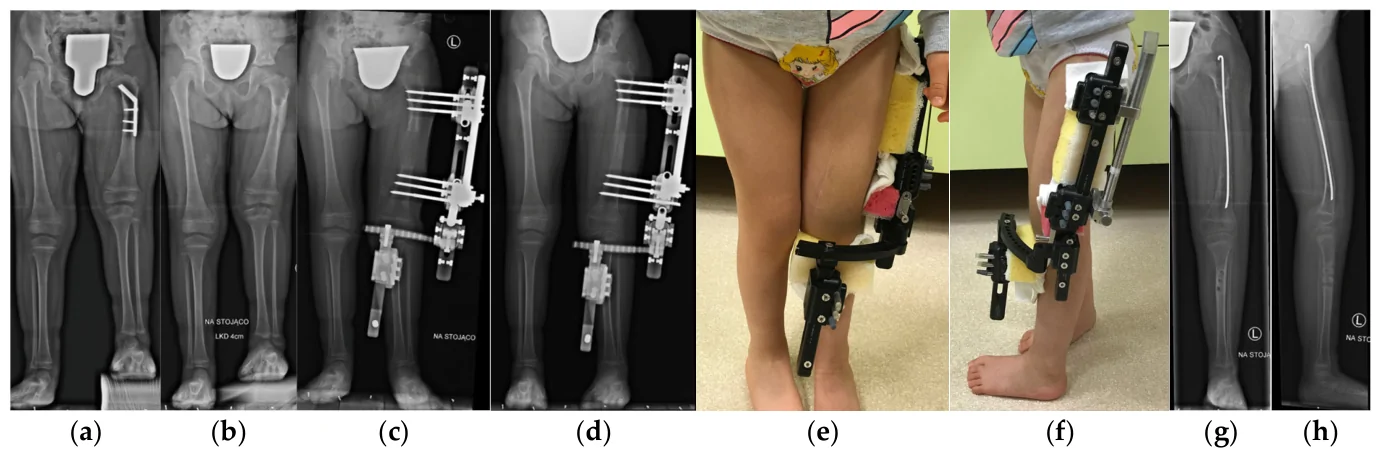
Radiographic and clinical views of left CFD type 1A: (a) standing radiograph after SUPERhip and SUPERknee procedures; (b) 5 cm shortening of the left femur before lengthening; (c) femoral lengthening using an MRS external fixator with a knee hinge; (d) consolidation phase after 5 cm femoral lengthening; (e,f) clinical views of the MRS external fixator; (g,h) prophylactic fixation with a Rush pin after MRS removal. L—left, LKD—left lower limb, NA STOJĄCO—standing position.
